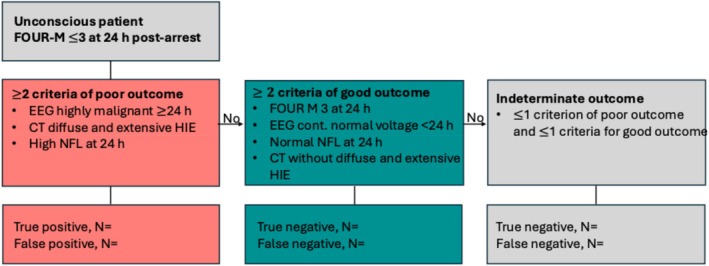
Study hypotheses. Patients unconscious at 24 h after cardiac arrest, defined as not being awake and following commands (FOUR‐M—score ≤ 3) will be evaluated according to the following criteria for likely poor (mRS 4–6) and good (mRS 0–3) outcome at 6 months. Criteria for poor outcome include electroencephalogram (EEG) highly malignant patterns (suppression or burst‐suppression) at ≥24 h, computed tomography (CT) with diffuse and extensive hypoxic‐ischaemic brain injury (HIE) ≥ 6 h after cardiac arrest or high blood levels of neurofilament light (NFL) at 24 h. Criteria for good outcome include localizing a painful stimulus on clinical neurological examination (FOUR‐M = 3), EEG with a continuous normal voltage background < 24 h, normal blood levels of NFL at 24 h or CT without diffuse and extensive HIE. Patients not fulfilling either criteria for likely poor outcome or good outcome likely will remain classified as indeterminate outcome. True positive: pathological criteria for poor outcome and poor functional outcome. False positive: pathological criteria for poor outcome and good functional outcome. True negative: good outcome and either criteria for good outcome or indeterminate outcome. False negative: poor outcome and either criteria for good outcome or indeterminate outcome. For patients with indeterminate outcome, the concordance and discordance between criteria of good and poor outcome prediction and the actual functional outcome will be presented in a separate table as described in the supplementary.
